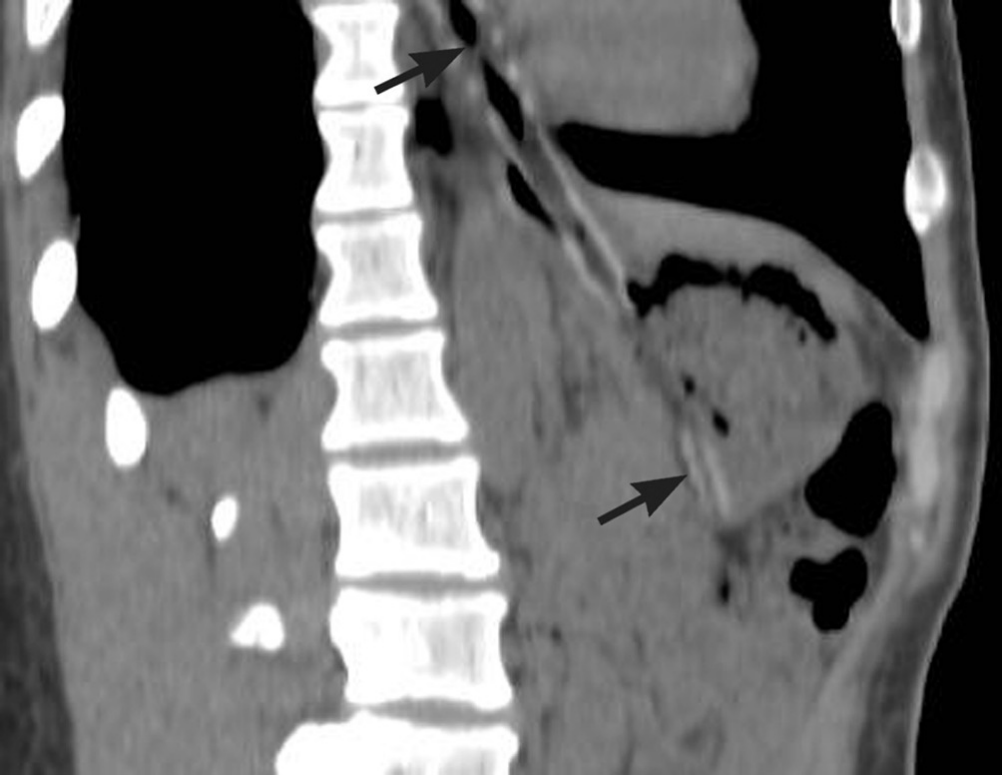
Thick maximum intensity projection CT image demonstrates a toothbrush (arrows) crossing the gastroesophageal junction. This long object (at least 17 cm) failed to descend into the stomach. As the patient feared endoscopy, which would normally be the initial approach for this foreign body removal, the toothbrush was removed surgically.
